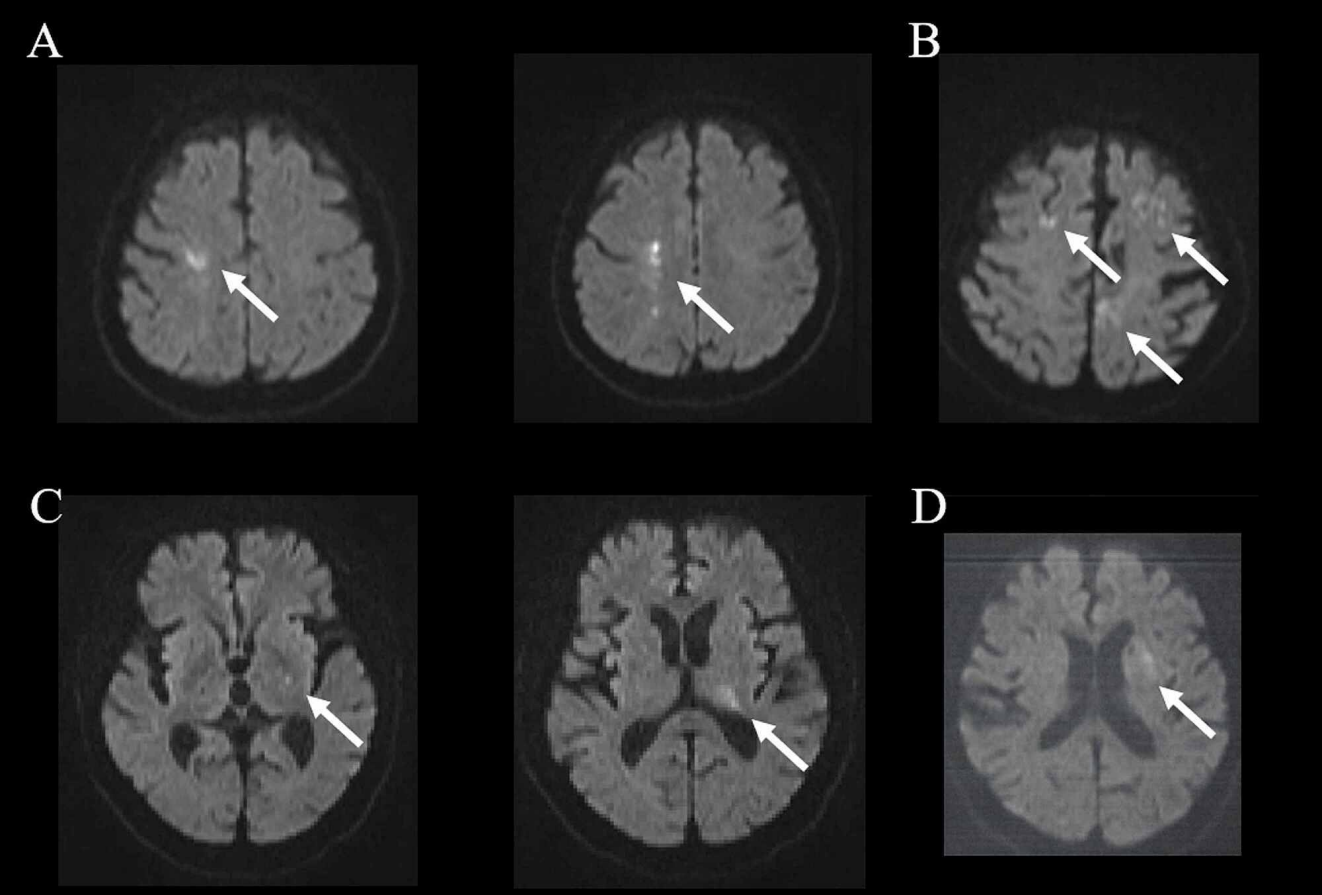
Postoperative DWI abnormalities. Postoperative DWI abnormalities (shown by arrows) in four patients (No. 3 in A, No. 7 in B, No. 21 in C and No. 33 in D).
